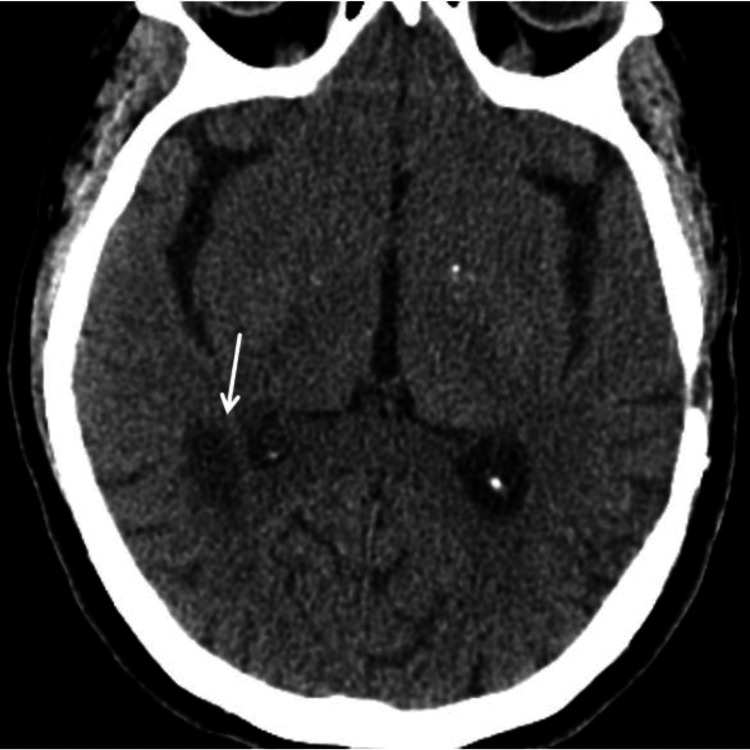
CT brain shows acute to subacute infarct in the right corona radiata and right posterior periventricular region (arrow).
